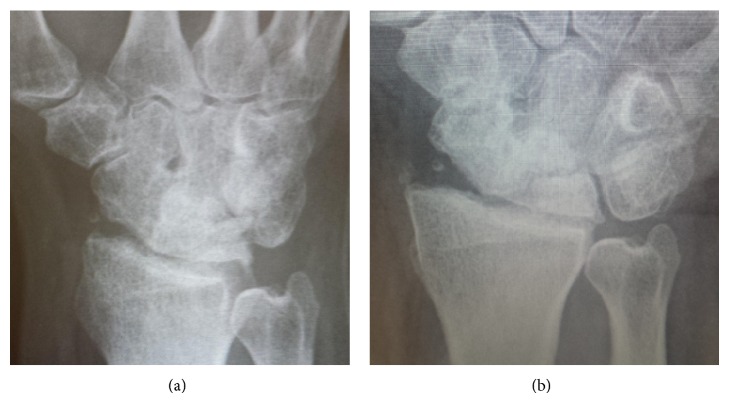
Wrist radiographs showing maintained joint space at 2 years.
